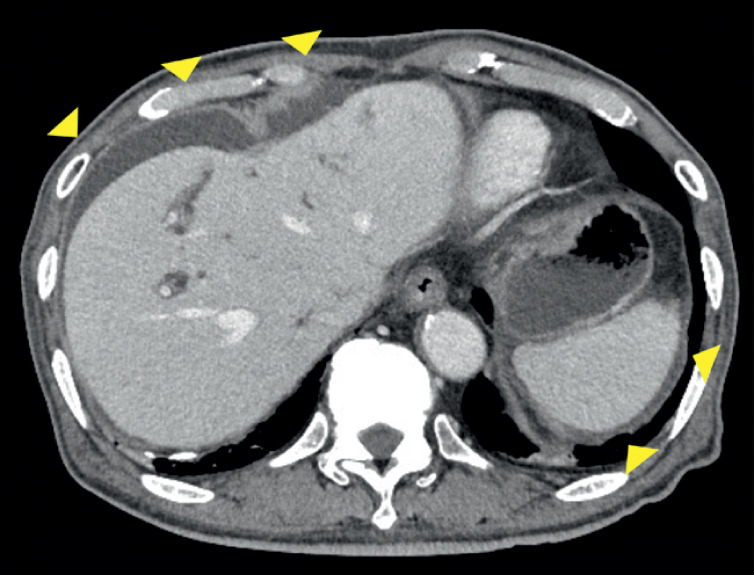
CT showed ascites around the liver, stomach, and spleen with the stent remaining in place, raising the suspicion of biliary peritonitis (arrowheads).
